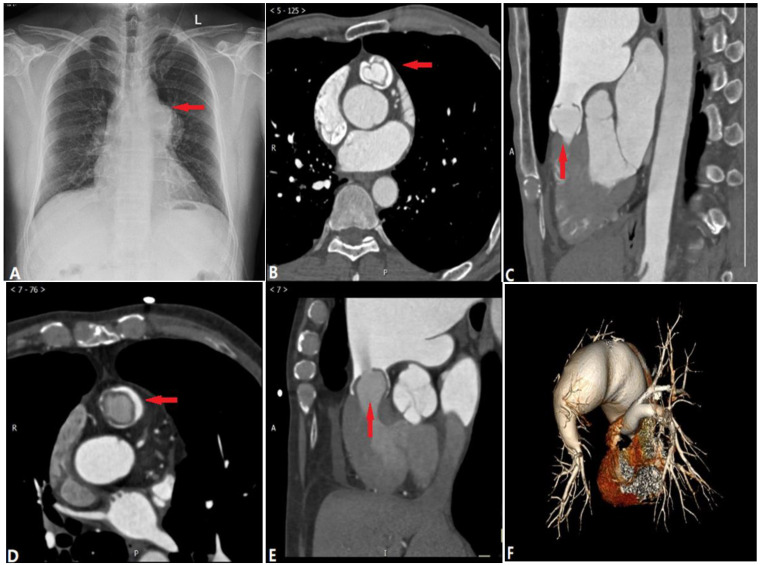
Pulmonary valvular stenosis—bicuspid pulmonary valve. (A): Chest radiograph shows the prominence of the left pulmonary artery, while the right pulmonary artery (arrow) and hilum appear normal. (B–E) belong to two different patients, but both show the stenotic bicuspid pulmonary valve (arrow) and post-stenotic dilation of the main and left pulmonary arteries, whereas the distal branches are relatively small. The jet of blood flow (arrow) is forced through the stenotic valve to the left pulmonary artery in (E). (F): volume-rendering of pulmonary arteries indicates the post-stenotic dilation of the main and left pulmonary arteries.
